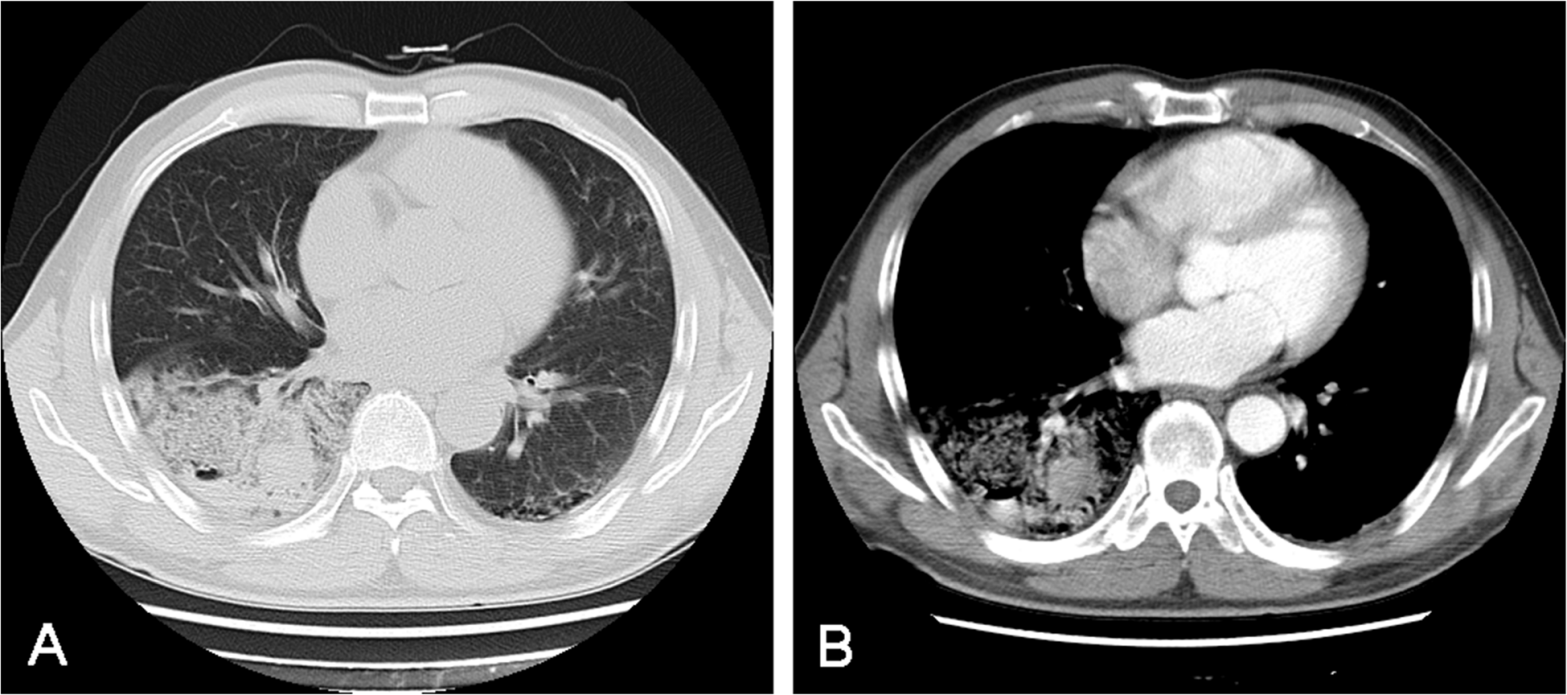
Chest computed tomography scan showed that parenchymal infiltrate with cystic lesion in the right lower lobe of lung
